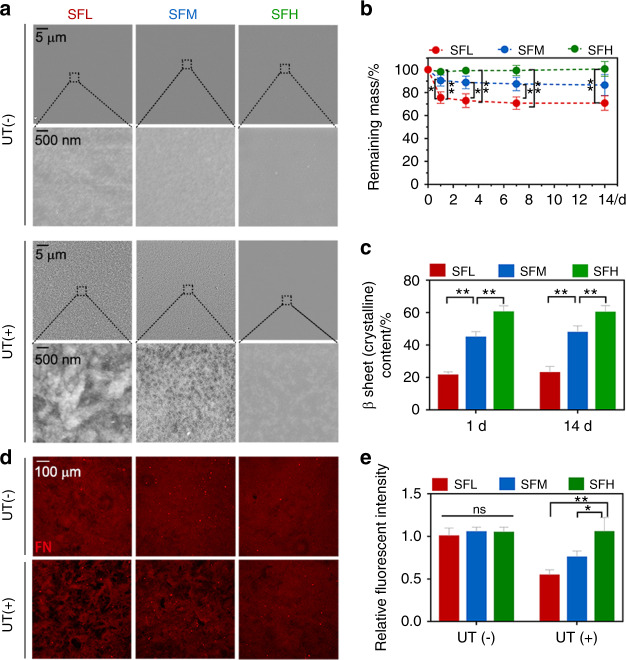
Interfacial stability between different SF substrates and adsorbed FN. a SEM images of different SF substrates after being immersed in PBS for 24 h, with and without ultrasonic treatment (UT). b, c Remaining mass (b) and β-sheet contents (c) of different SF substrates immersed in PBS with UT treatment at specific time points. d, e Immunofluorescence images (d) and the quantitative analysis (e) of adsorbed FN on different SF substrates after being immersed in FN solution for 24 h, with and without UT treatment. Error bars represent one standard deviation. (P* < 0.05 and *P* < 0.01)
